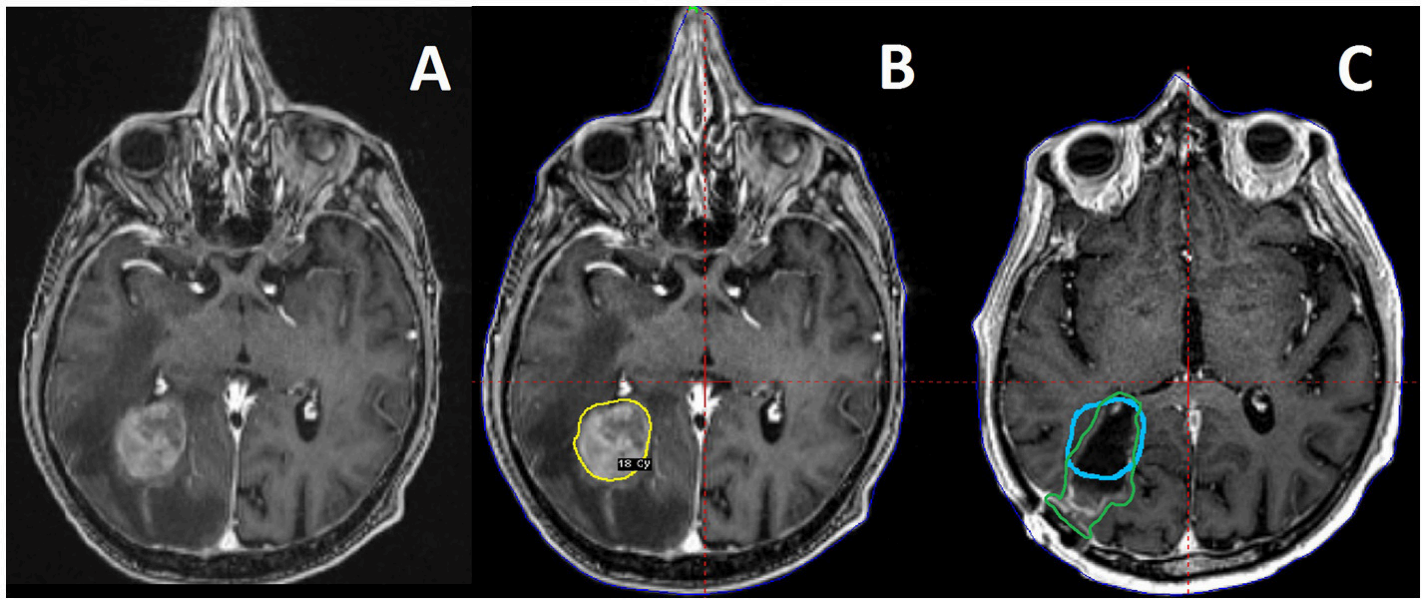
A 73 year old male patient with metastatic soft tissue sarcoma and three brain metastases, one large right occipital metastasis with associated edema (A), as well as smaller tumors in the right motor strip and left temporal lobe (not pictured). He was treated with preoperative SRS (B) to 18 Gy to the 50% isodose line (20 Gy to the other, smaller tumors) followed by surgical resection of the occipital tumor the next day. Also pictured is a 3 month follow up MRI with the preoperative target depicted in blue, compared to the postoperative target depicted in green as per consensus guidelines (C), demonstrating the change in the tumor cavity geometry after resection.
